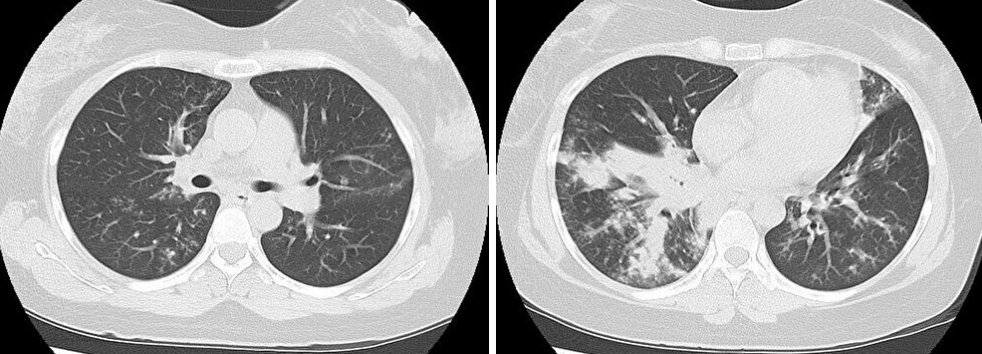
Chest computed tomography performed at the time of admission, showing infiltrates in both lungs.
